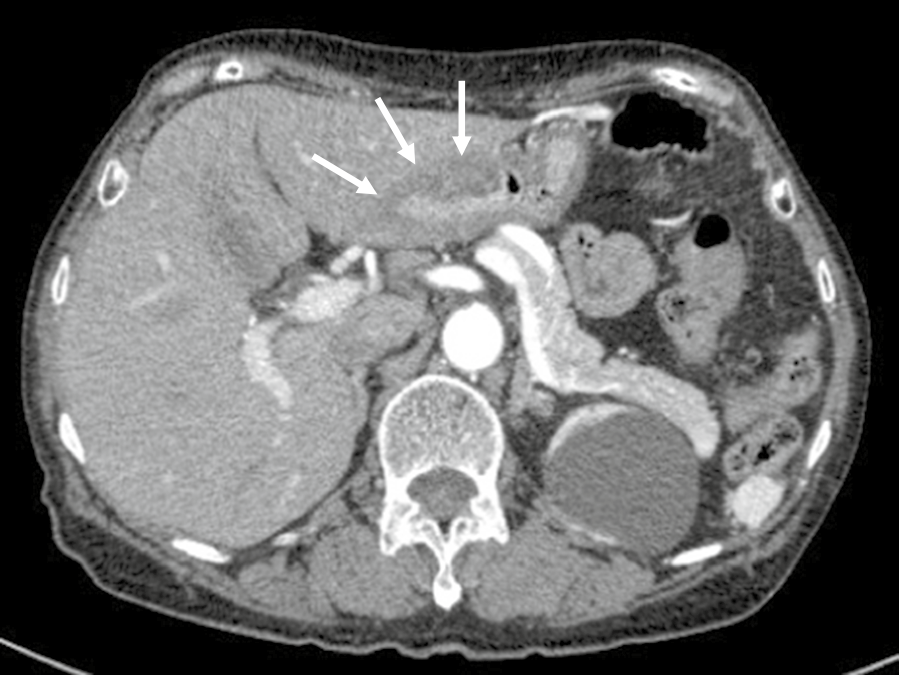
Contrast-enhanced computed tomography image. Increased wall thickness of the gastric antrum was observed (arrows)
